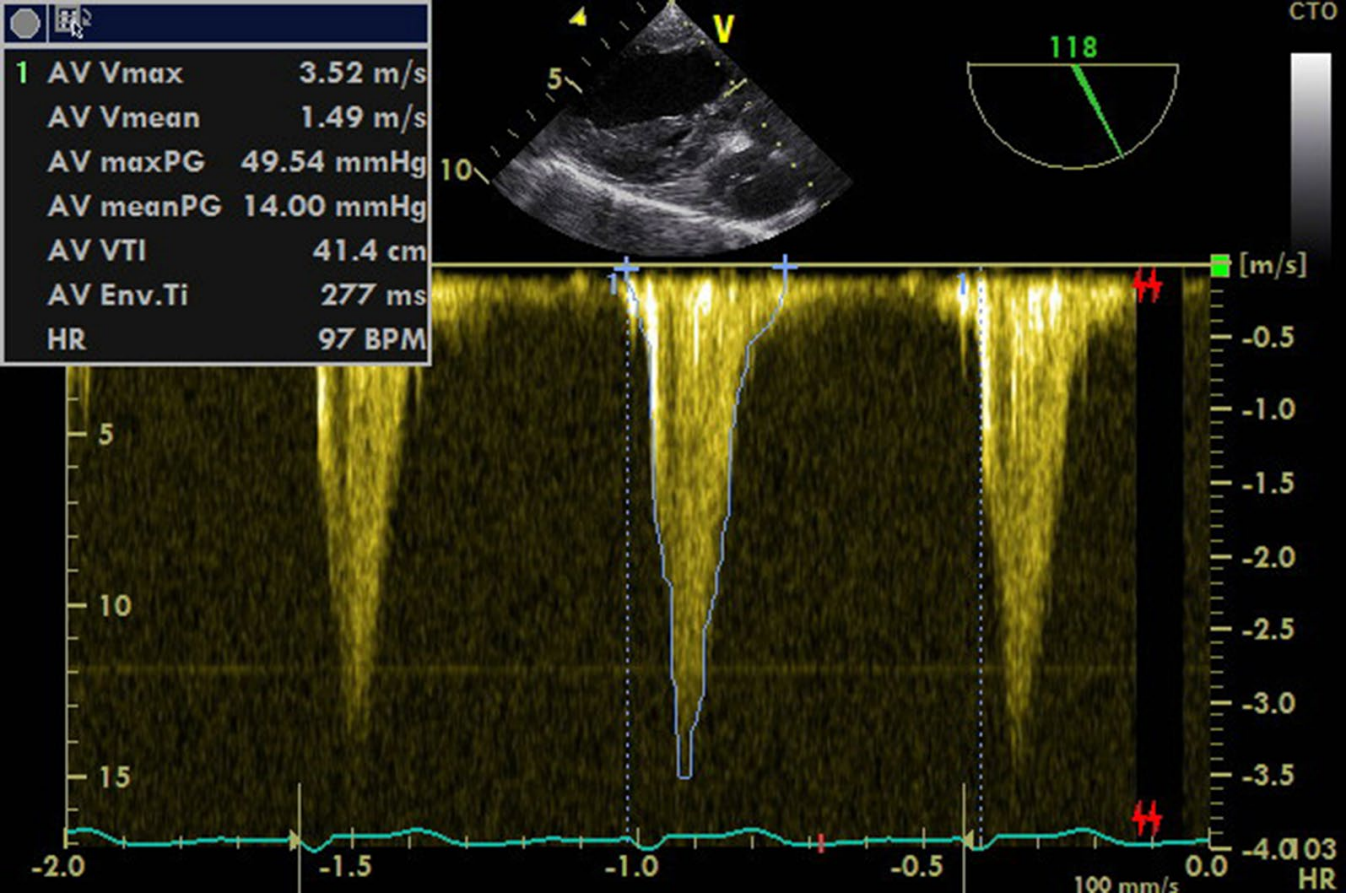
Transgastric long-axis view at 118°. Continuous-wave Doppler shows 3.5 m/second velocity across left ventricular outflow tract with indicating moderate obstruction
